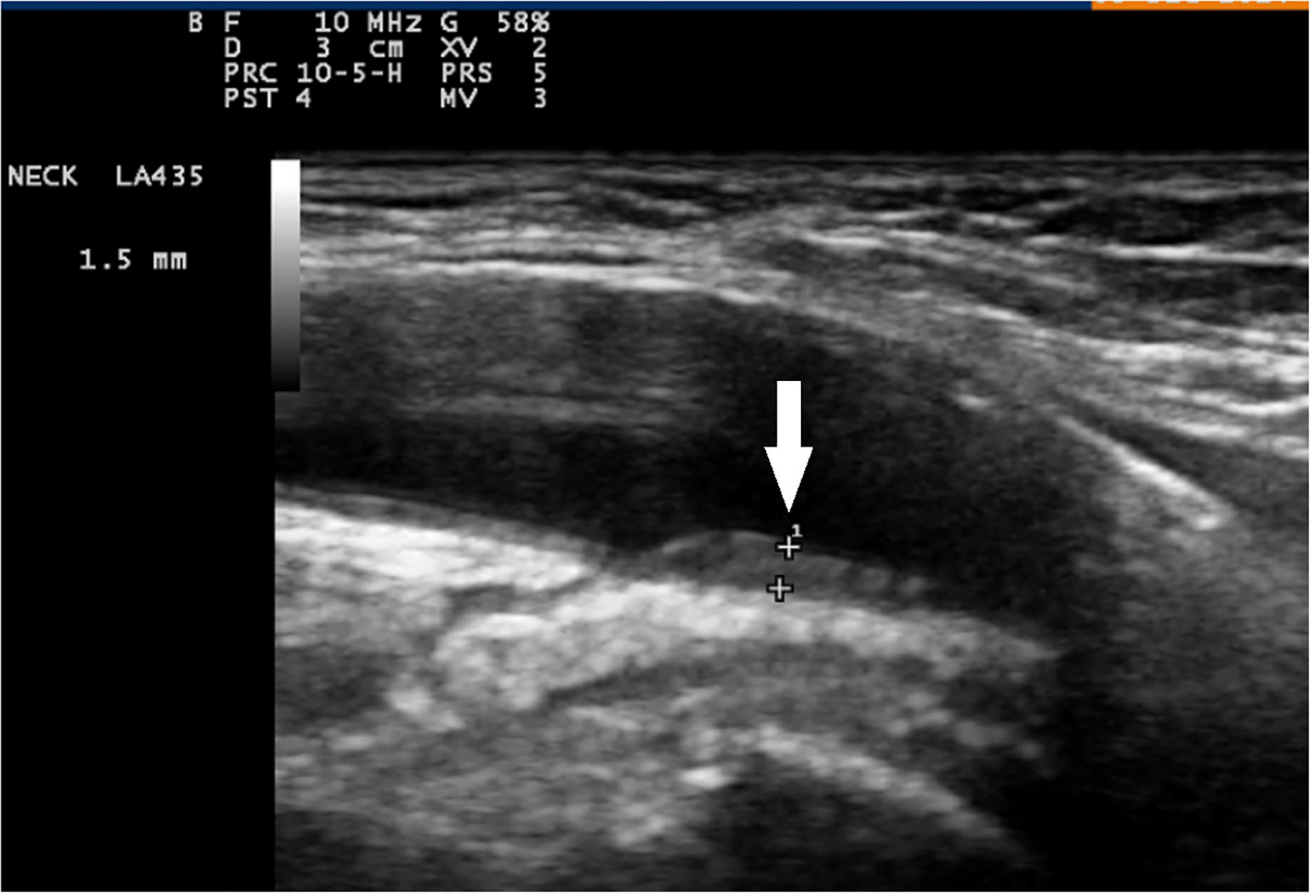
Color duplex sonography of axillary artery and longitudinal plane. Signs of vasculitis in an axillary artery (arrow) sliding down to a normal intima-media in the brachial artery (slope sign).
